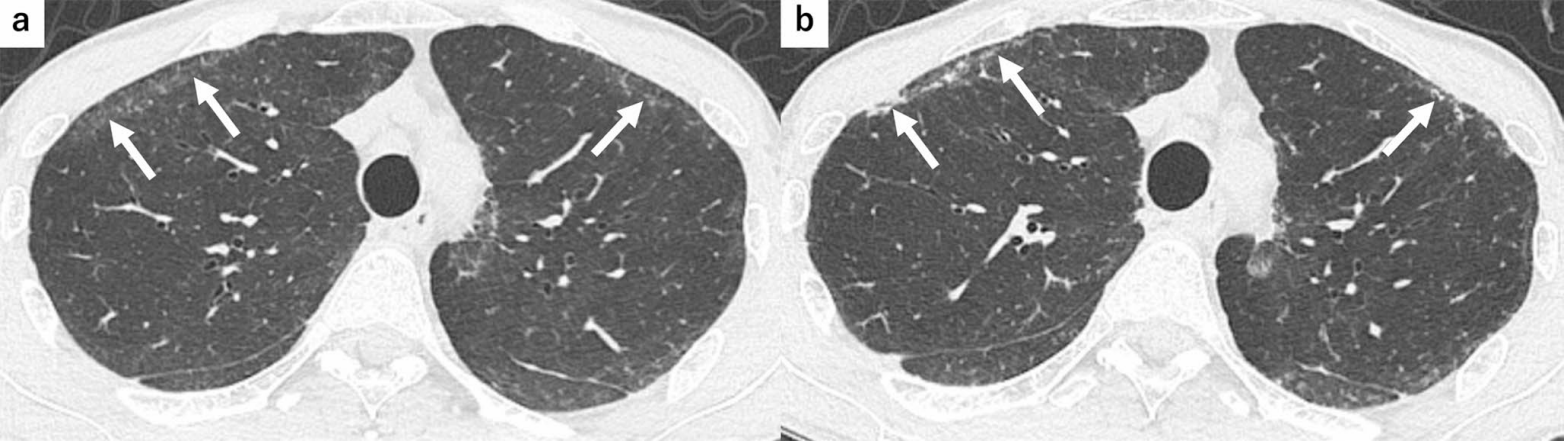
A 57-year-old man with FANO. a Pre-biopsy unenhanced axial chest HRCT shows FANO in the bilateral anterior upper lobes (arrows). b Follow-up HRCT at 2 years demonstrates progression with coalescence into subpleural curvilinear opacities and reticulation (arrows). *FANO* faint amorphous nodular opacity, *HRCT* high-resolution CT
